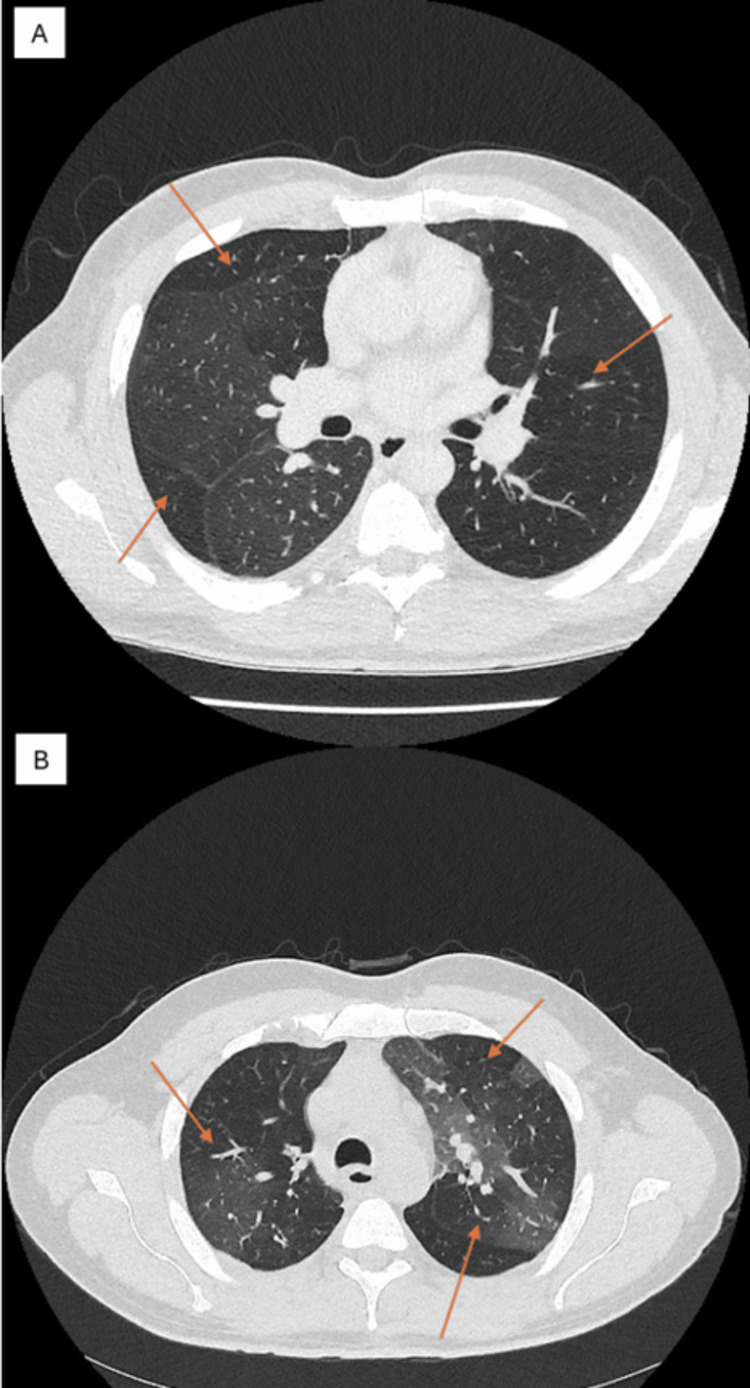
CT at diagnosis of bronchiolitis obliterans, with arrows showing air trapping and mosaic attenuation
